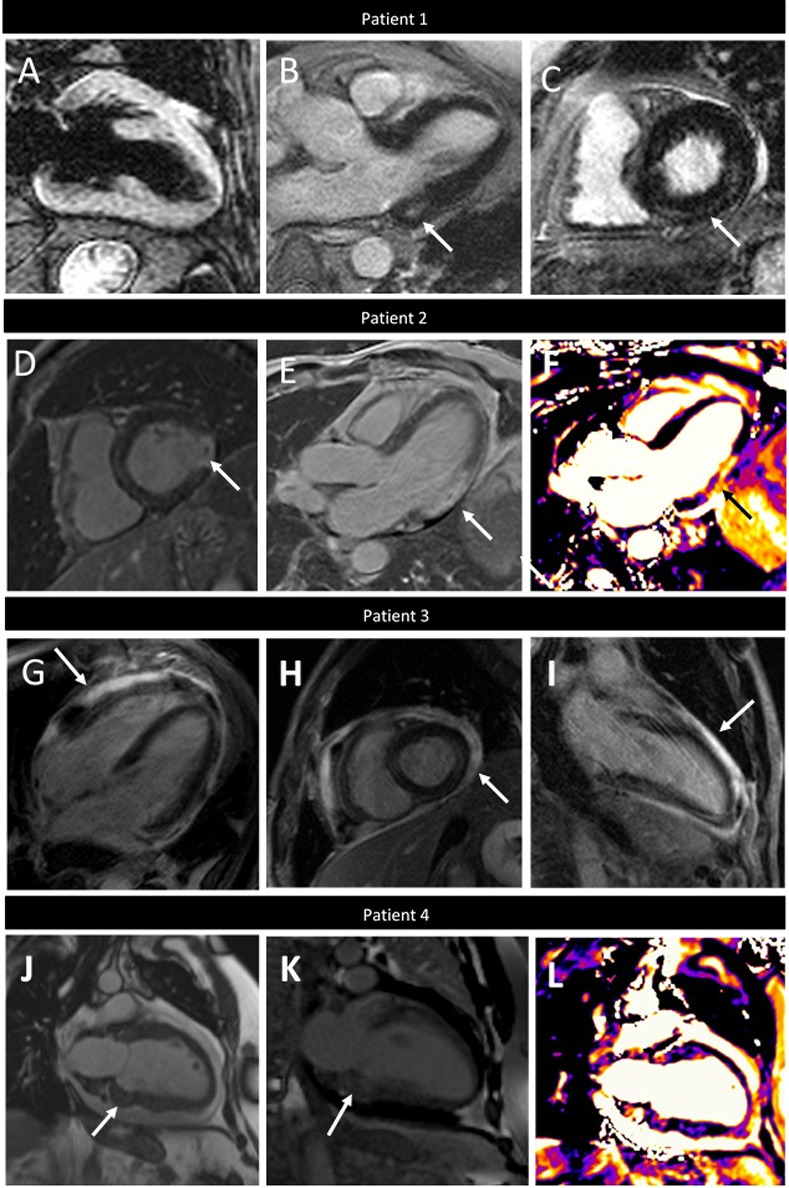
Varying CMR presentations of Immune Checkpoint Inhibitor (ICI) associated cardiotoxicity. Patient 1. Myocarditis with elevated T2-weighted signal (T2 ratio 2.2) (A) three chamber (B, white arrow) and short axis (C, white arrow) LGE imaging showing mid-myocardial LGE of basal inferior and inferoseptal walls. Patient 2. Acute myocardial infarction showing transmural LGE of the inferolateral wall with microvascular obstruction (D and E, white arrows) and corresponding increased signal on T2W-mapping (F, black arrow). Patient 3. Myopericarditis showing pericardial thickening and LGE on four chamber (G), short axis (H) and two chamber (I). Patient 4. Metastatic tumors within the basal inferior myocardium on two-chamber cine imaging (J, white arrow) with corresponding LGE (I, arrow) and edema on T2W imaging (J, black arrow).
